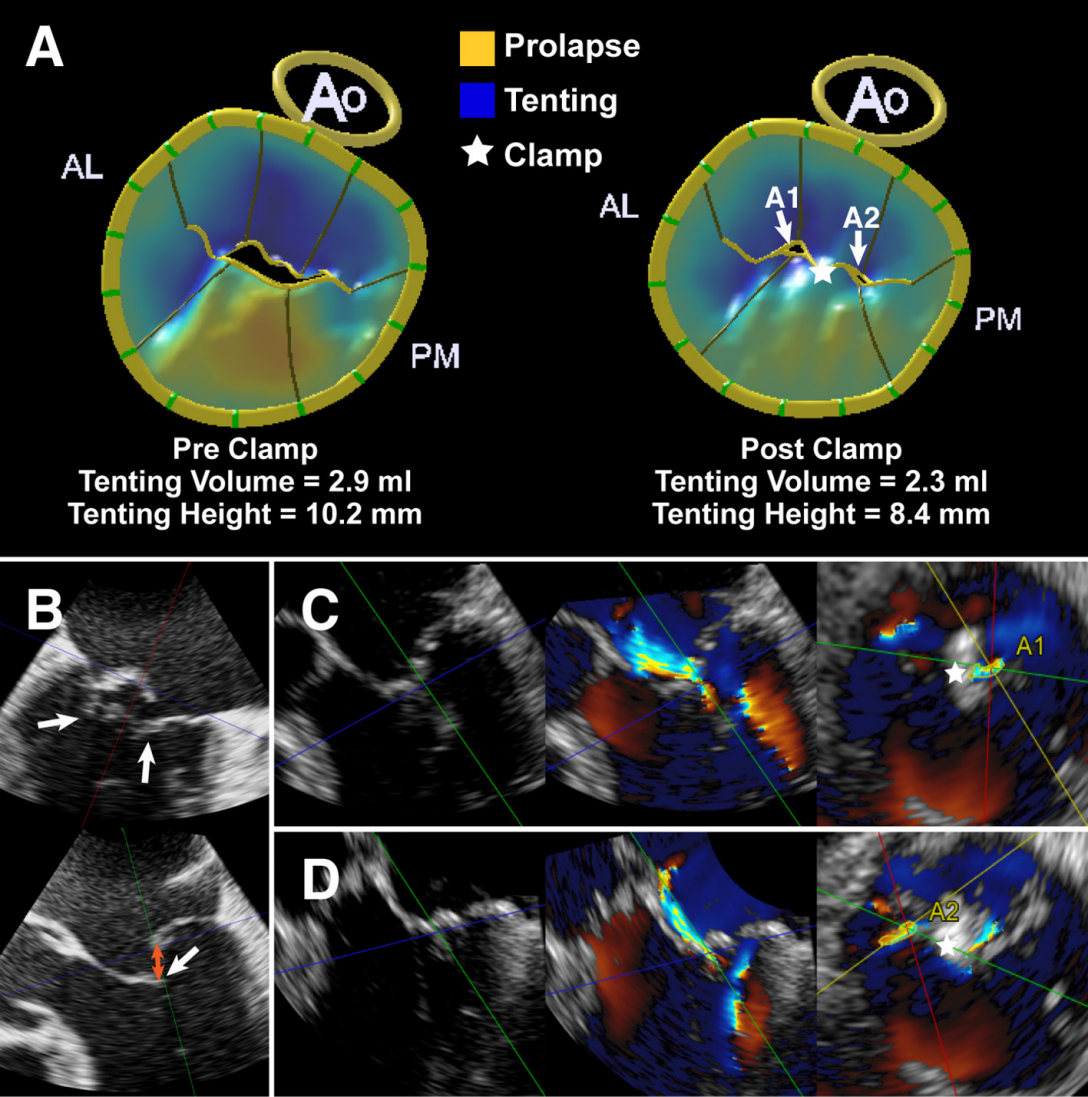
A typical case with P2 prolapse/flail and secondary bileaflet tethering. (A) Left: MV modeling from MVN analysis vividly demonstrating a prolapsing P2 scallop (shaded yellow) and tented scallops (shaded blue). Right: MV modeling showing the residual tenting volume (shaded blue) and residual regurgitant orifice (A1 and A2). (B) MV commissural view and long-axis view derived by multiplanar reconstruction (MPR) from real-time 3D dataset showing preoperative P2 prolapse/flail and leaflet tethering (white arrow). Orange arrow indicates the flail/coaptation gap. (C,D) MPR images derived from 3D Color Doppler dataset showing leaflet tethering with residual regurgitant jets after ValveClamp implantation. The total vena contracta area (VCA) was as follows: VCA_A1_ + VCA_A2_ = 0.16 cm^2^ + 0.12 cm^2^ = 0.28 cm^2^. Left: color compress images; Middle: color Doppler images; Right: VCA measurements. Asterisks indicate the implanted clamp.
